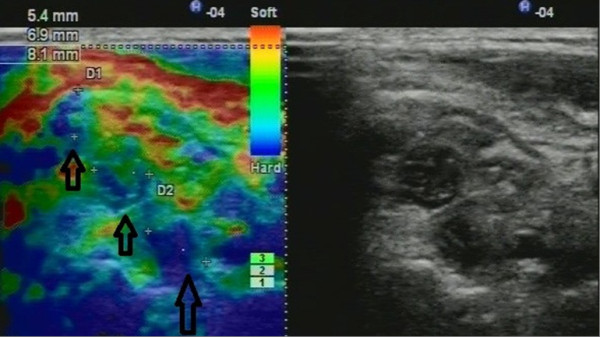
Ultrasound of brachial plexus neuropathy. Arrows indicate enlarged (swollen) brachial plexus trunks up to 8 mm (contralateral trunks were about 3 mm). Sonoelastography (left) indicates rigid tissues of trunks (colored blue). Gray-scale ultrasonogram (right).
